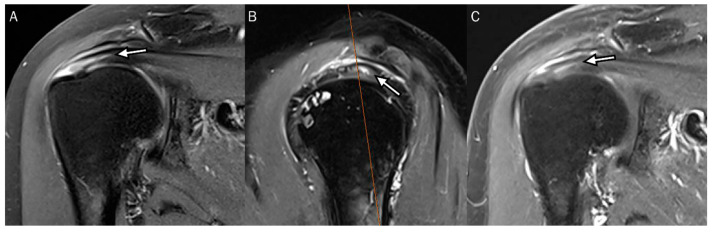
Enhancement of the synovial intraarticular tissue at the delaminated tear. (A) Oblique coronal fat-suppressed T2-weighted image and (B) coronal fat-suppressed T2-weighted image demonstrate the articular-delaminated full-thickness tear of the supraspinatus tendon with a visible cleavage (arrow). (C) The oblique coronal fat-suppressed T1-weighted enhanced image shows the synovial enhancement at the delamination (arrow).
